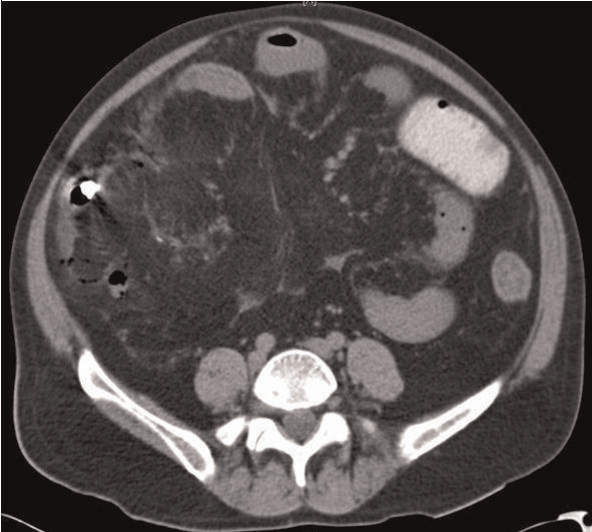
Small bowel perforation caused by capsule endoscope. Contrast-enhanced CT scan of the abdomen taken on admission showing loculated free air, fat stranding and a capsule endoscope near the terminal ileum
